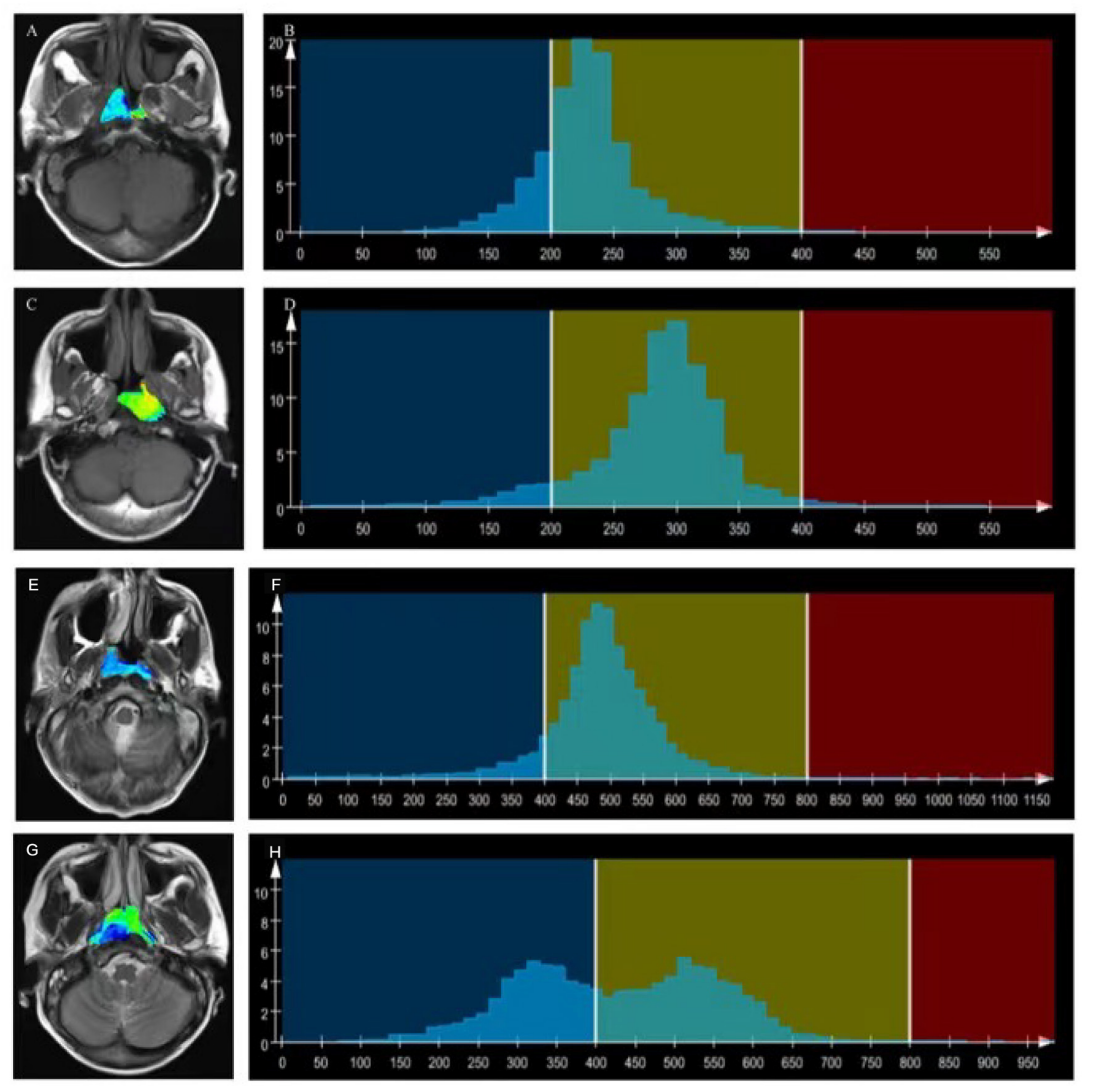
T1WI overlaid with color maps (A,C) and histograms of whole-tumor using T1WI maps (B,D). A T1WI histogram from (A) male patient with EBV DNA NPC showed higher T1WI_*Skewness*_ (T1WI_*Skewness*_ = 0.737). (B) than that of a male patient with EBV DNA-positive NPC (T1WI_*Skewness*_ = 0.435). (D) T2WI) overlaid with color maps (E,G) and histograms of whole-tumor using T2WI maps (F,H). A T2WI histogram from a female patient with EBV DNA-negative NPC showed obviously higher T2WI_*Kurtosis*_ (T2WI_*Kurtosis*_ = 9.004) (F) than that of a male patient with EBV DNA-positive NPC (T2WI_*Skewness*_ = −0.958) (H). T1WI, T1-weighted imaging; EBV, Epstein–Barr virus; NPC, nasopharyngeal carcinoma, T2WI, T2-weighted imaging.
